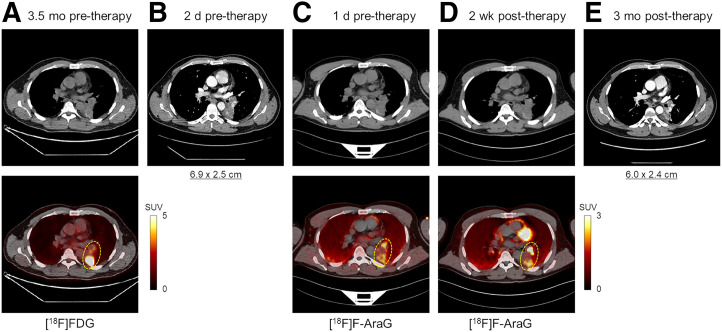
Transverse slices through LLL mass of subject 5, comparing initial [^18^F]FDG PET/CT 3.5 mo before therapy (A), contrast-enhanced CT 2 d before therapy (B), [^18^F]F-AraG PET/CT 1 d before therapy (C), [^18^F]F-AraG PET/CT 2 wk after therapy (D), and contrast-enhanced CT 3 mo after therapy (E).
